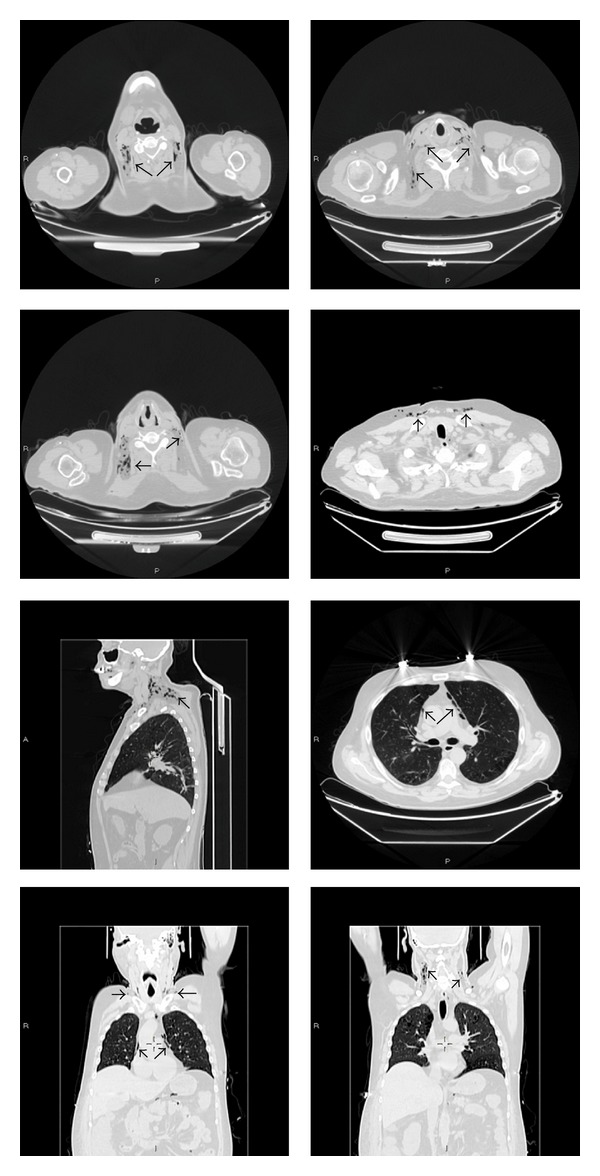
Whole body CT scan revealed free gas in the mediastinum and subcutaneous tissue, dissecting soft tissues and muscles from the maxillary to the sternocleidomastoid muscle and the sternoclavicular joint bilaterally. Free gas was also evident in correspondence of the dorsal and pectoral soft tissues (black arrows).
